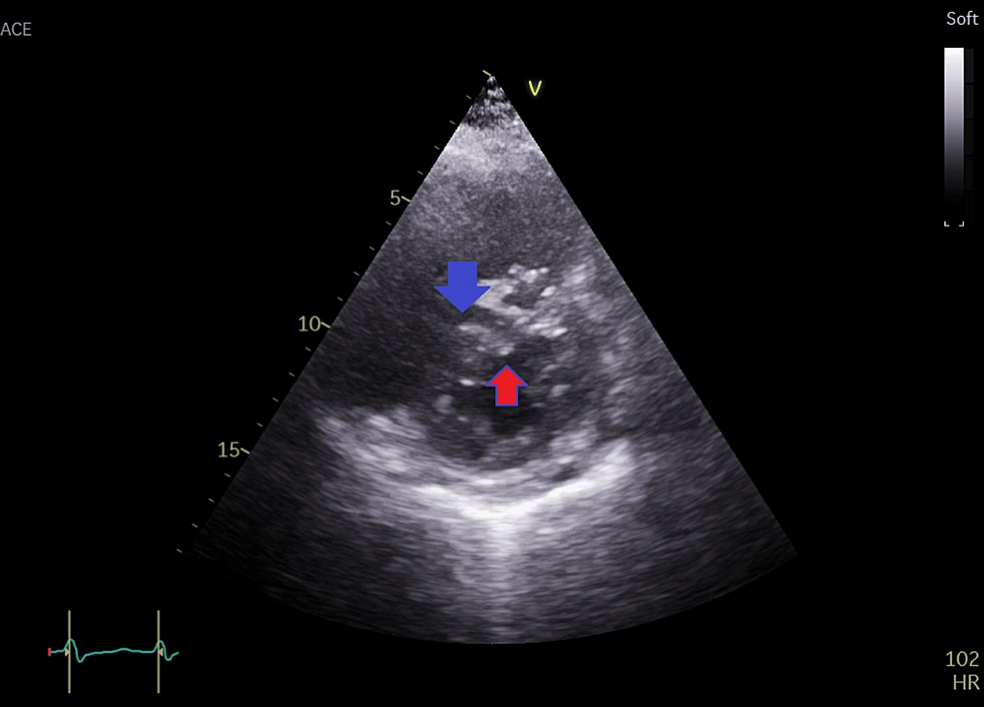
Left parasternal short-axis view of the left and right ventricles showing dilated right ventricle (blue arrow) with right ventricular volume overload: D-shaped left ventricle (red arrow) in diastole
